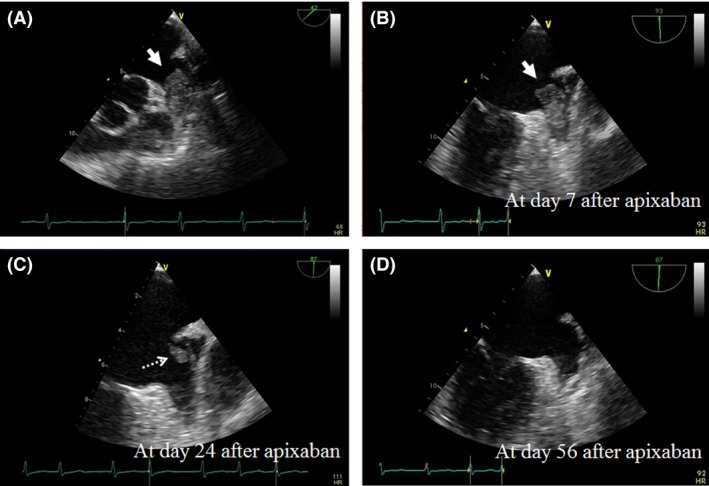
Transesophageal echocardiogram (TEE). (A) Left atrial appendage (LAA) thrombus (white arrow) was observed at first presentation. (B) No change of thrombus of LAA on day 7 after apixaban treatment. (C) Reduction in thrombus of LAA (white dotted arrow) on day 24 after apixaban treatment. (D) Complete resolution of LAA thrombus was achieved on day 56 after apixaban treatment.
